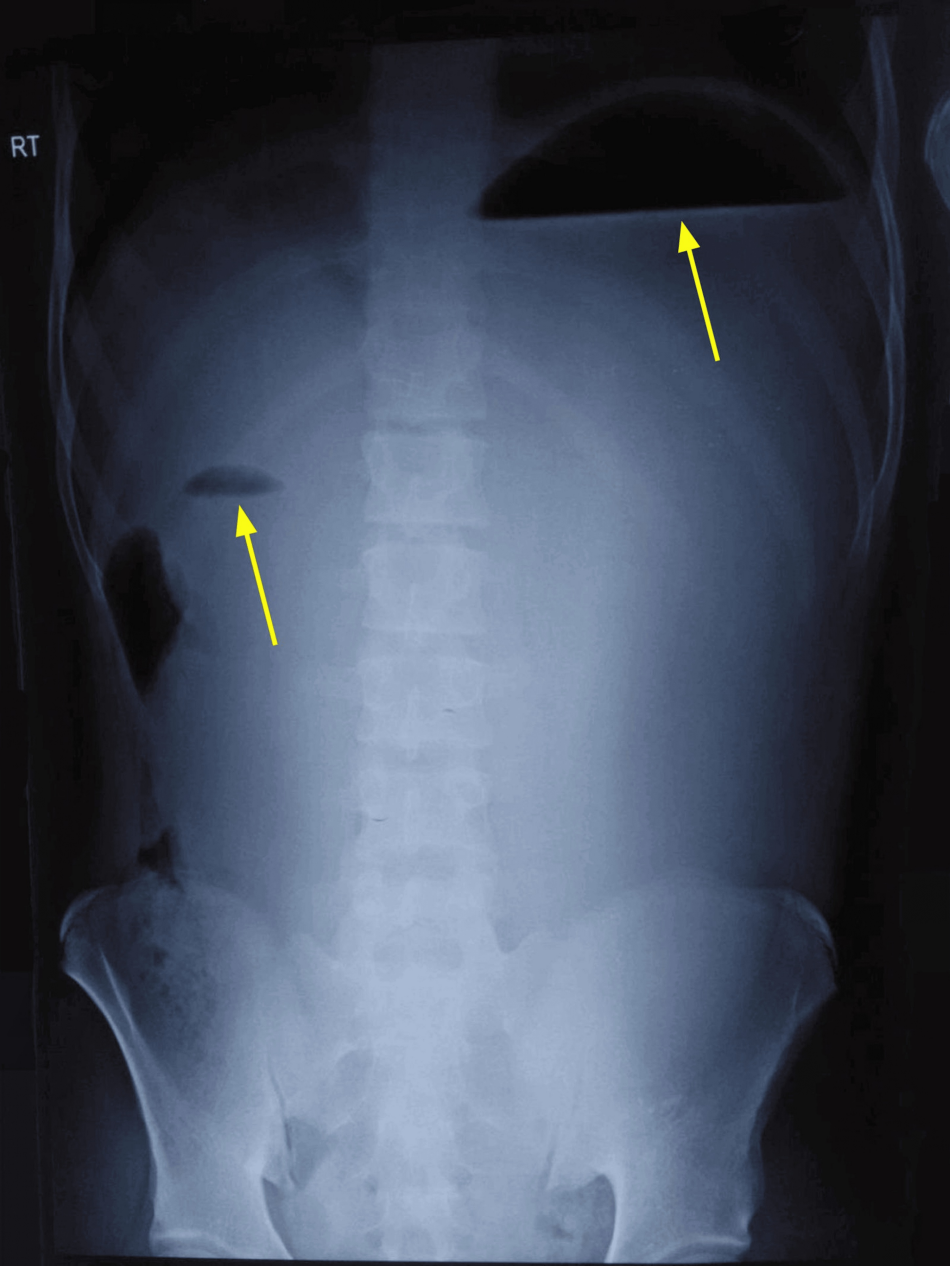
Abdominal X-ray revealing a potential “double bubble” sign suggestive of duodenal obstruction (yellow arrows)
